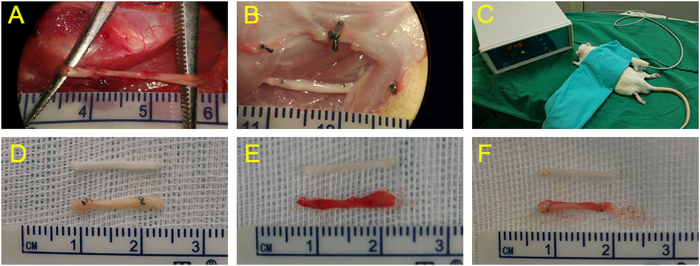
In the control group, 12 weeks after transplantation, an autograft sciatic nerve was 10-mmlong and swelling, adhesion to the surrounding tissue (A). In the low-dose group, an autograft sciatic nerve was 10-mmlong and with less nervous tissue inflammation (B). Experimental apparatus for applying low-intensity pulsed ultrasound. The transducer was placed on the right leg operational area skin via ultrasound gel (C). General view of different group sciatic nerve: low-dose group (D), high-dose group (E) and control group (F).
